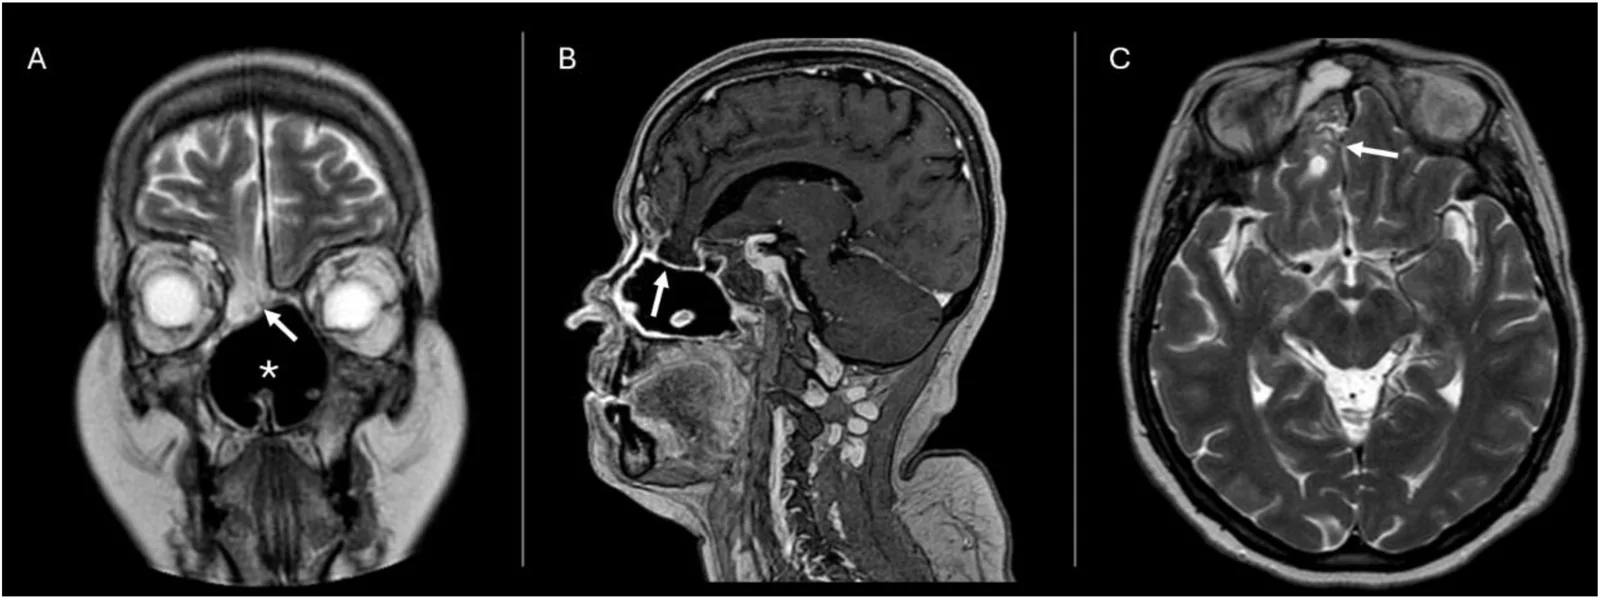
Brain magnetic resonance images. (A) Coronal T2-weighted image demonstrating extensive erosive involvement of the nasal cavity (*), with a right ethmoidal roof defect and herniation of the orbitofrontal gyri, which demonstrates T2 hyperintensity (arrow). (B) Contrast-enhanced sagittal T1-weighted image shows pachymeningeal enhancement within the hernia sac (arrow). (C) Axial T2-weighted image shows cortical thinning and adjacent white matter T2 hyperintensity consistent with encephalomalacia and gliosis.
